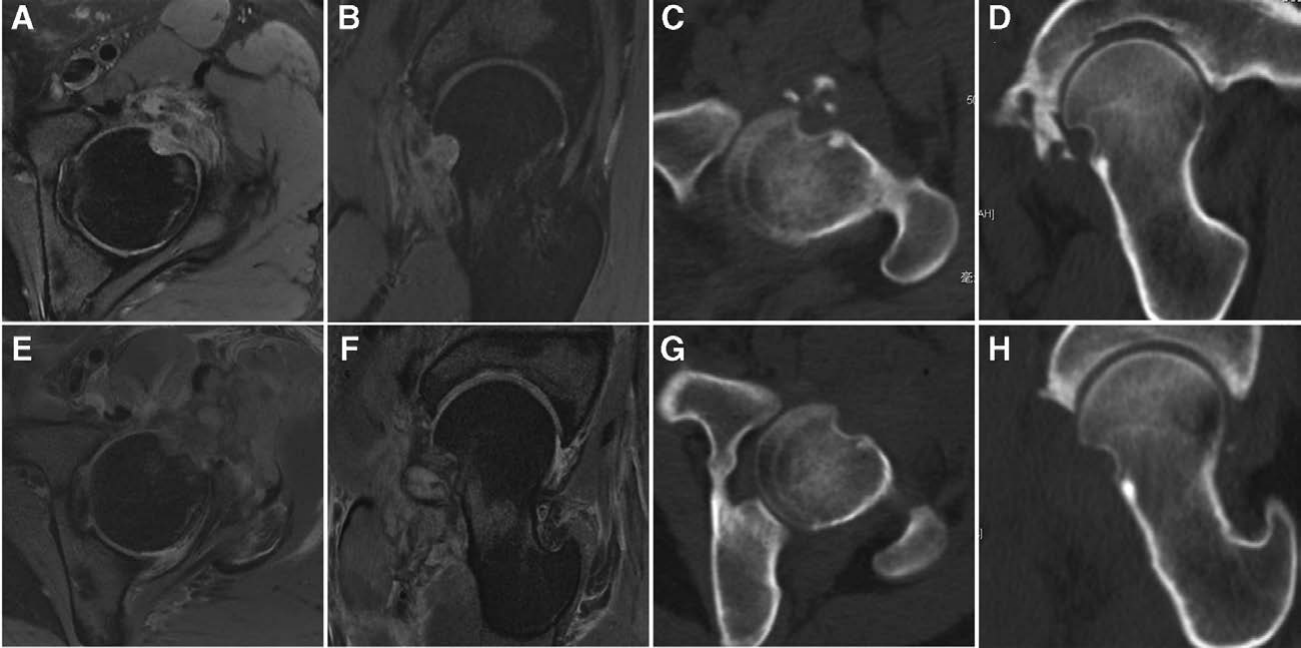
(A, B) Preoperative axial and oblique sagittal MRI showed soft tissue mass in the femoral head neck junction. (C, D) Preoperative axial and oblique sagittal CT showed adjacent bone defect and ossification. (E, F) Postoperative axial and oblique sagittal MRI showed total excision of the mass. (G, H) Postoperative axial and oblique sagittal CT showed total excision of the mass. CT = computed tomography, MRI = magnetic resonance images.
